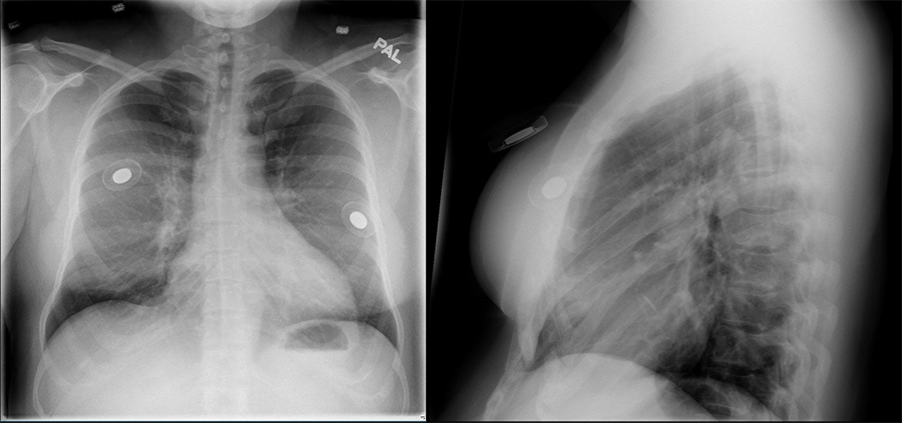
Patient 2. A chest radiograph revealed two dense ring-like objects in the regions of the breasts, which were the metallic injection ports of the breast tissue expanders.
